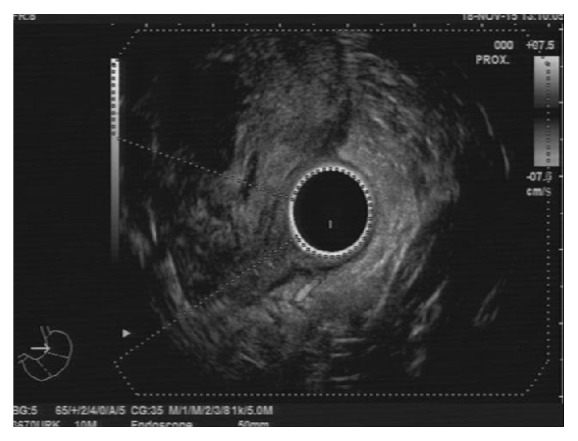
Endoscopic ultrasonography revealed avascular a heteroechoic submucosal mass at the posterolateral wall of the duodenum.
